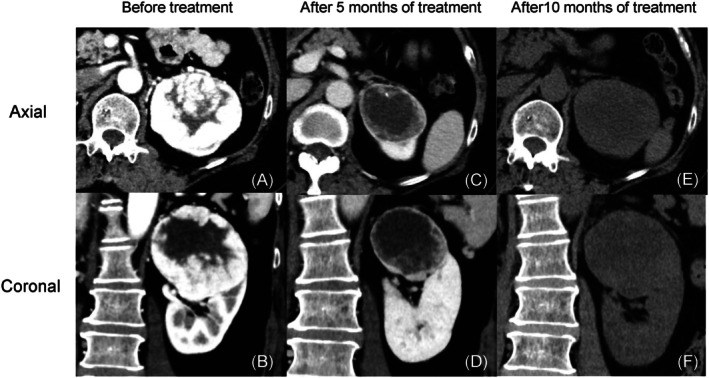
Contrast‐enhanced computed tomography images of the left kidney. (A) Axial view before treatment. (B) Coronal view before treatment. (C) Axial view after 5 months of treatment. (D) Coronal view after 5 months of treatment. (E) Axial view after 10 months of treatment. (F) Coronal view after 10 months of treatment.
